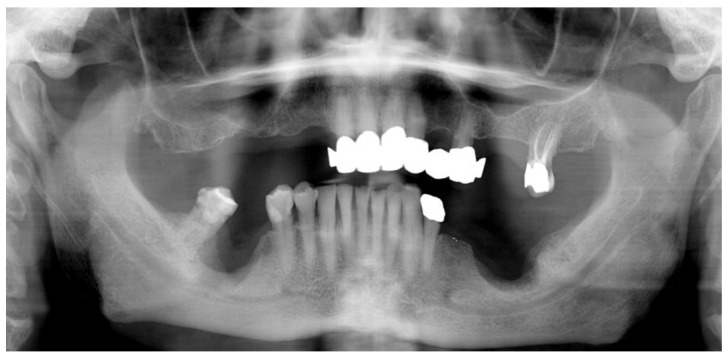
The 3-month postoperative panoramic radiograph.
